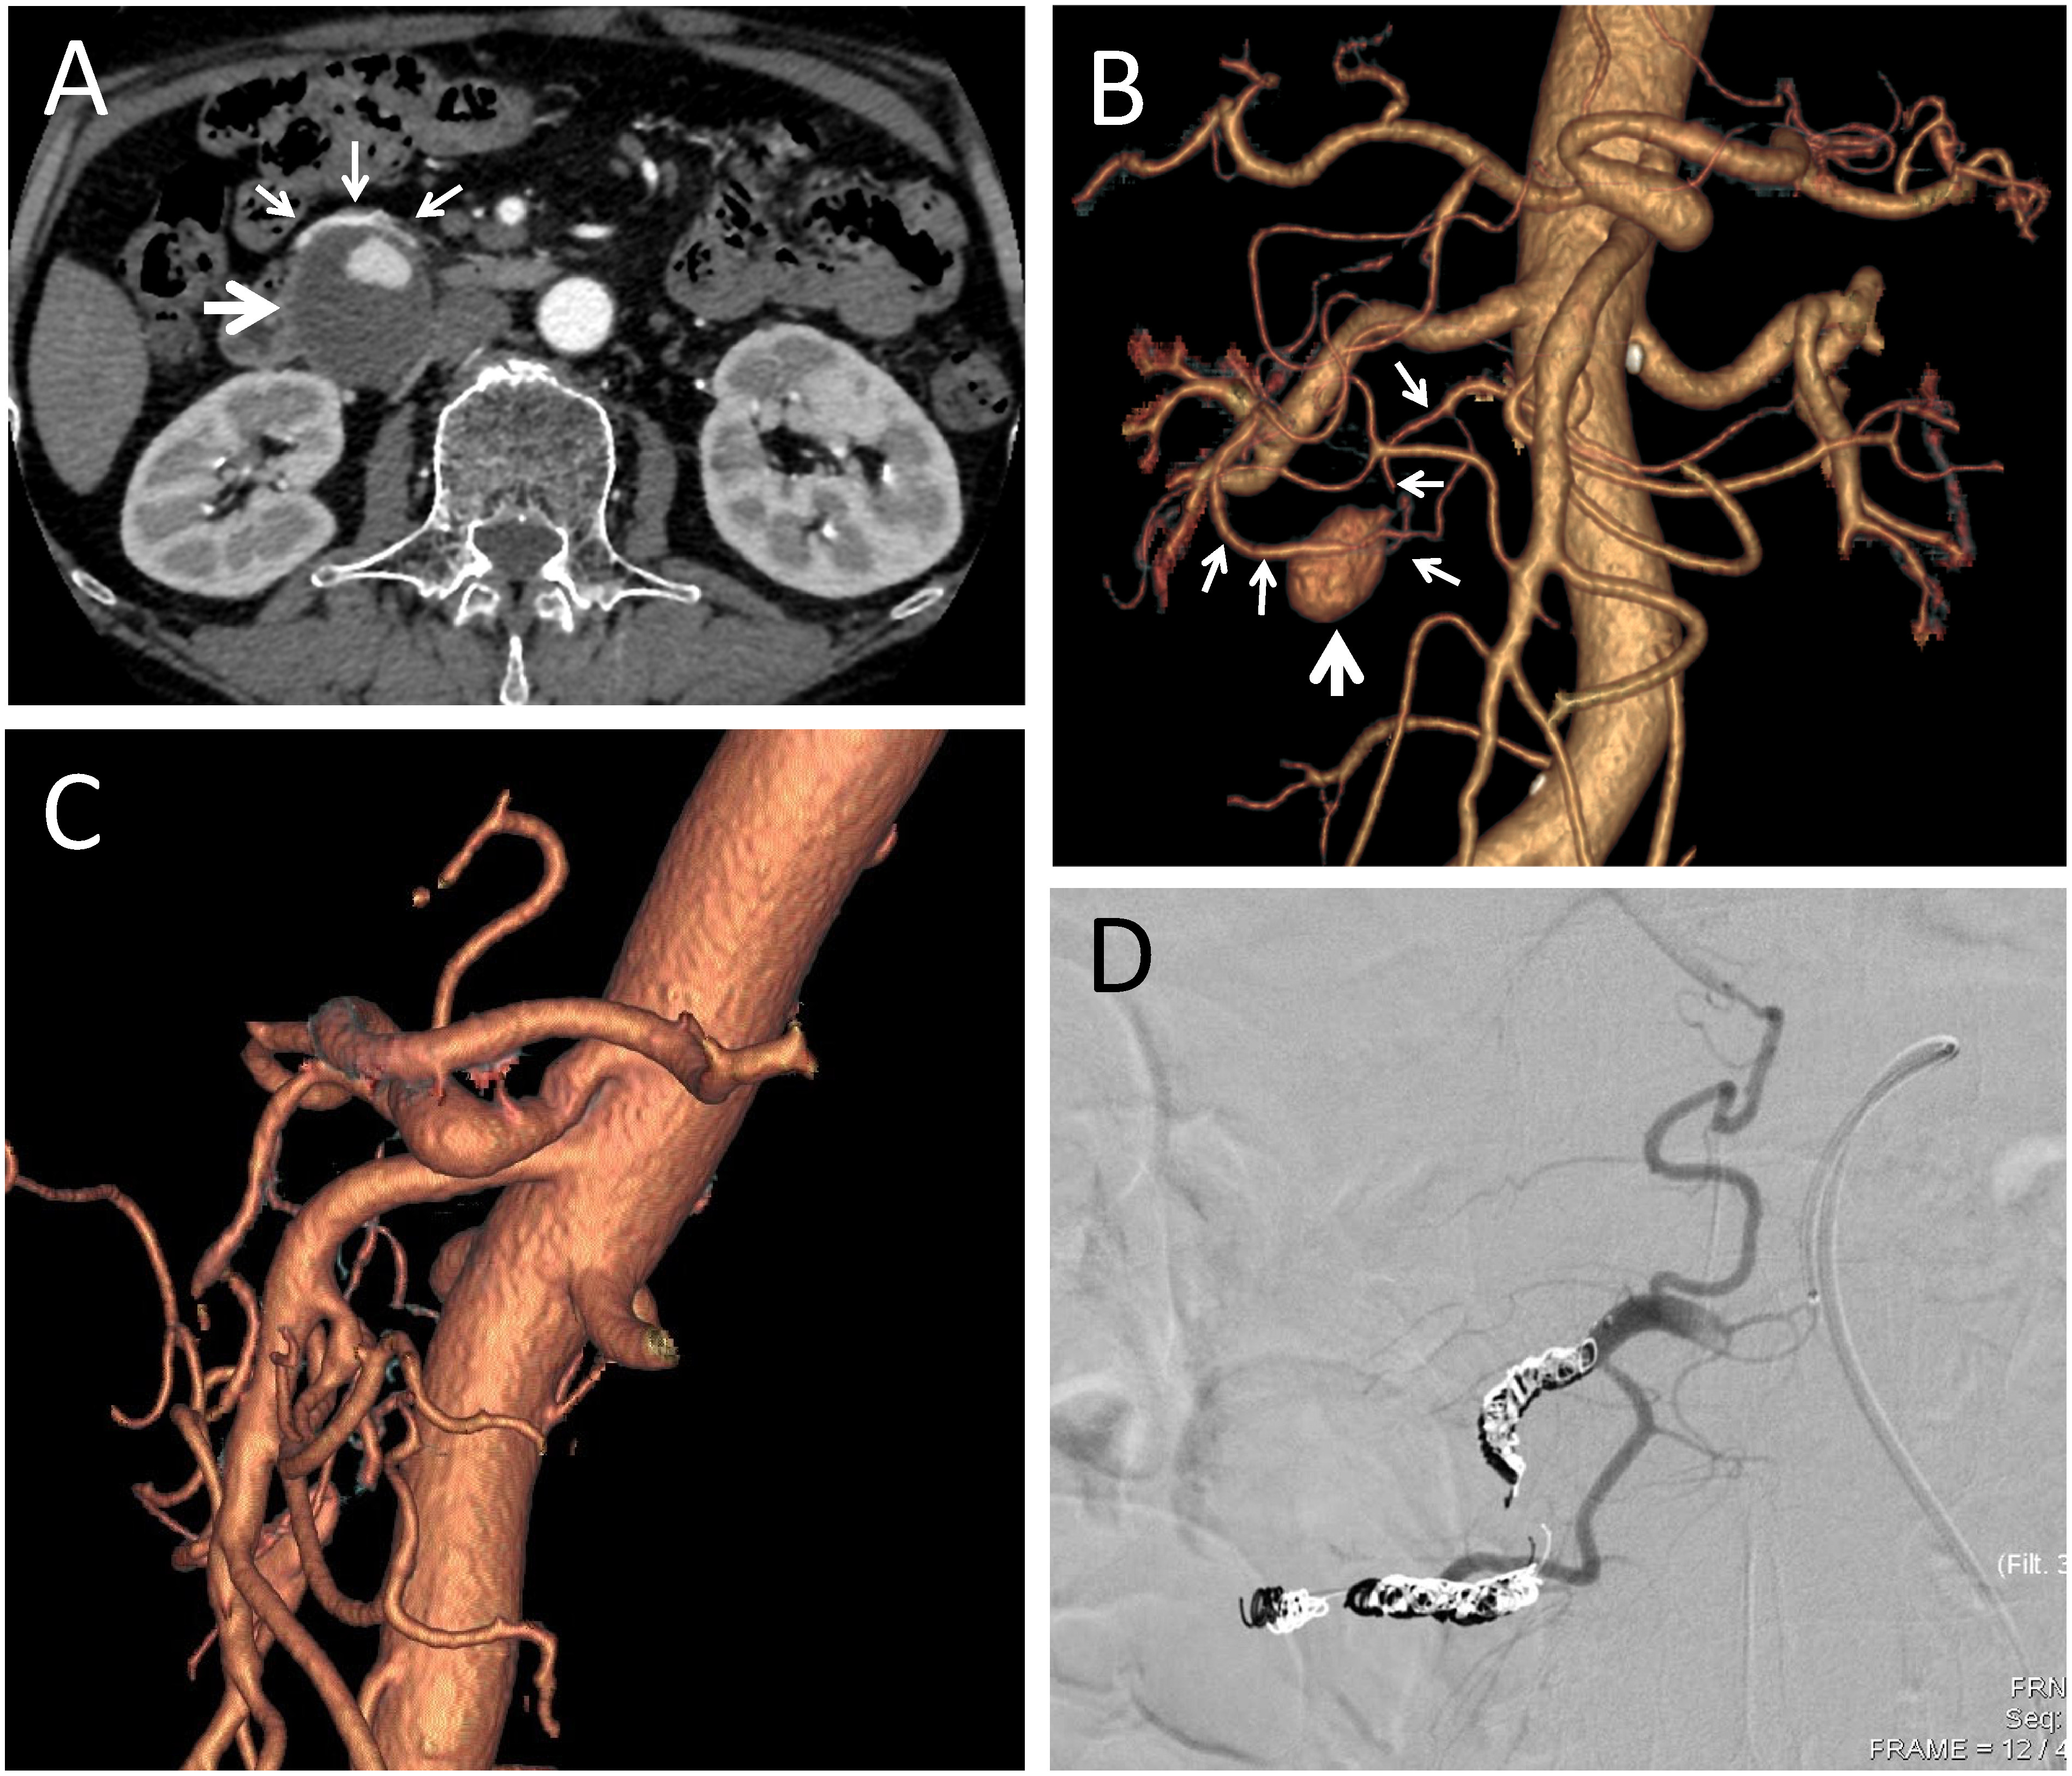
Fig. 1 Images for patient 1. (A) Preoperative computed tomography (CT) slice demonstrating the pancreaticoduodenal artery aneurysm (large arrow). (B) Three-dimensional (3D)-CT image of the aneurysm (large arrow) and the adjacent vascular anatomy. (C) Lateral 3D-CT image demonstrating no obstructive lesion at the celiac artery axis. (D) Angiographic image after coil embolization of the anterior superior pancreaticoduodenal artery (ASPDA). The aneurysm is no longer visible. Small arrows: ASPDA.
